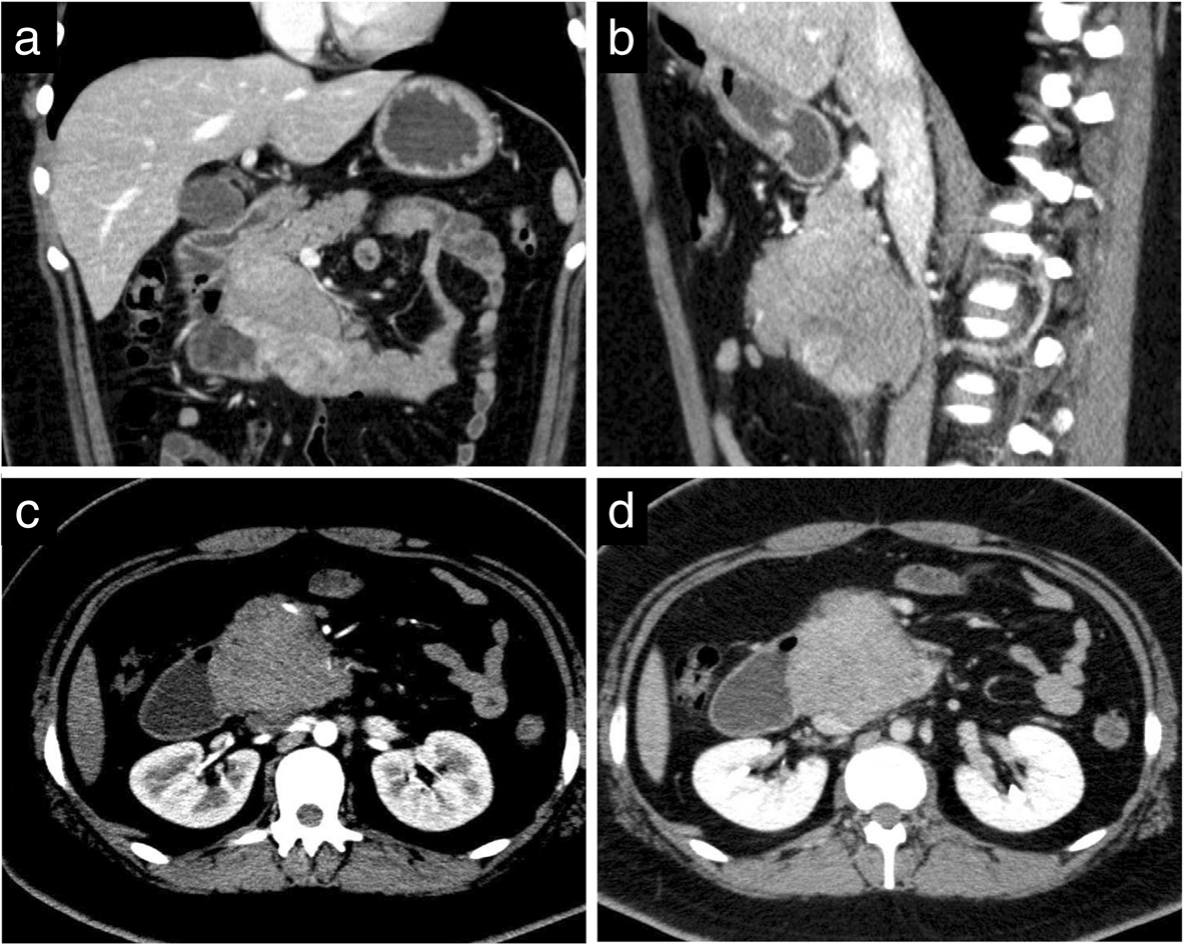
Contrast-enhanced CT of the abdomen. a The tumor was located mainly in the uncinate process of the pancreas and invaded the duodenum. The oral side of the duodenum was expanded. b The tumor excluded the inferior vena cava with no evidence of direct invasion. c The arterial phase: the tumor completely enclosed some intestinal branches. d The portal phase: there was no evidence of portal invasion
